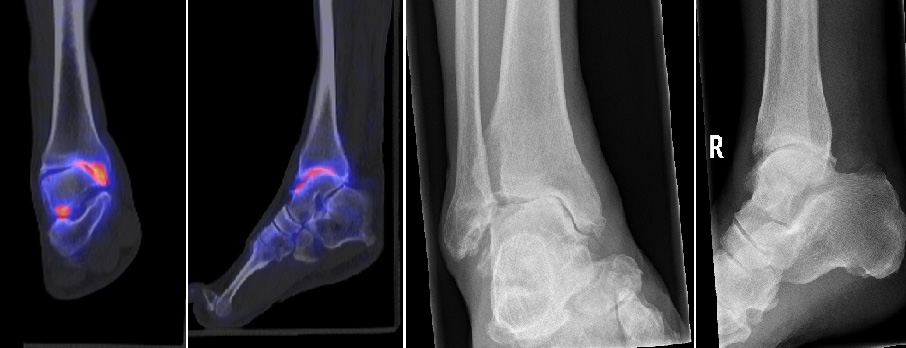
This patient is a 77-year-old male with no history of trauma. Since one and a half years, he has progressive pain and swelling of his right ankle after hiking and playing tennis. On examination, there is a mild varus axis and mildly swollen ankle. Although talocalcaneal is stiff, it is pain free on passive motion. There is mild pain while applying pressure subtalar on the medial side. The X-ray showed OA of the ankle with a asymmetrical joint line and possible subtalar OA. The diagnosis initially was talocrural and subtalar OA. SPECT/CT showed only OA and focal uptake in the sinus tarsi. The treatment plan changed from ultrasound-guided injection to an ankle arthrodesis.
